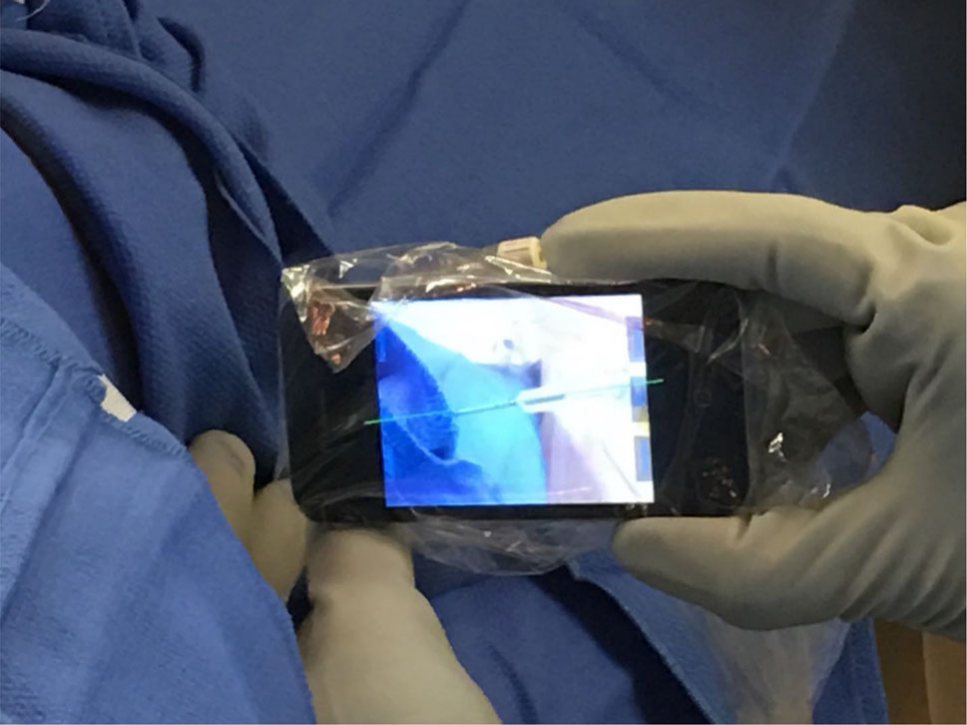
Smartphone with custom application for needle angle selection demonstrated in a real patient biopsy. Smartphone with custom application for imaging the needle and reproducing the angle of superimposed (*green*) needle insertion pathway for a CT-guided biopsy. The pathway was pre-selected with a procedural CT scan and the phone was calibrated to the CT table prior to placement in a sterile translucent bag. The actual needle resides beyond the smartphone, and the shaft and hub are seen superimposed on the needle path plan (*green line*). The application used the smartphone camera and gyroscope, and interfaces with the procedural CT scan DICOM images via Bluetooth
